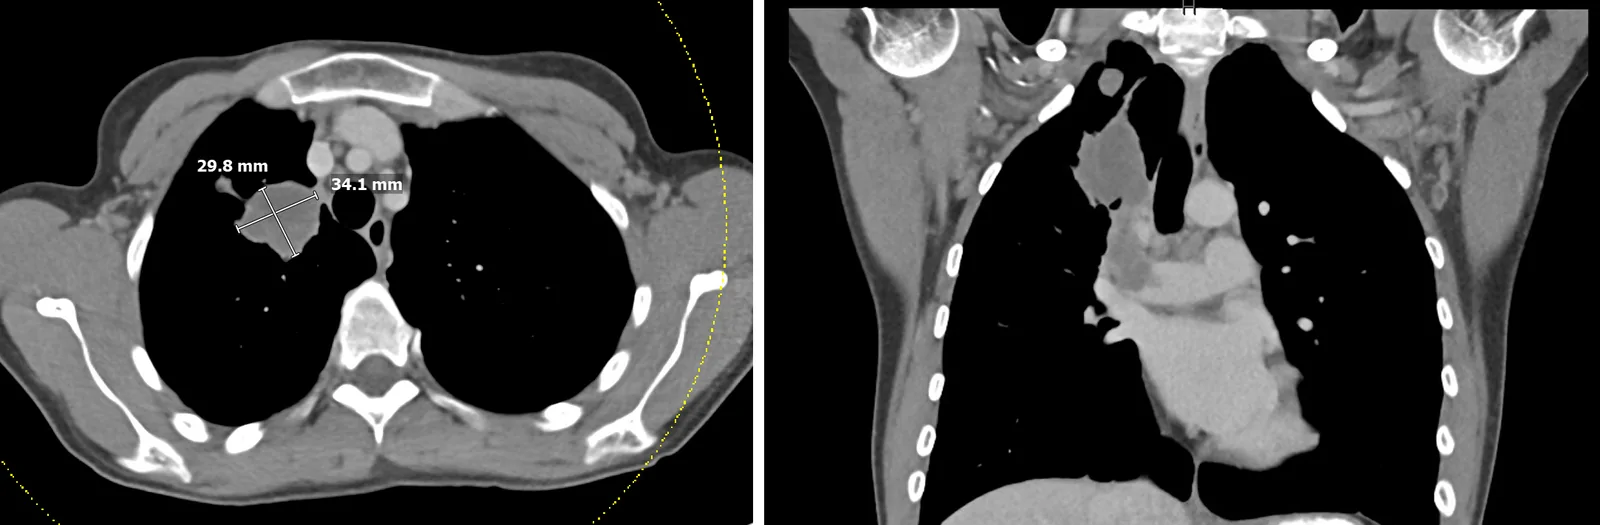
CT performed after three cycles of neoadjuvant pembrolizumab, two days prior to pneumonectomy and pulmonary artery reconstruction.
